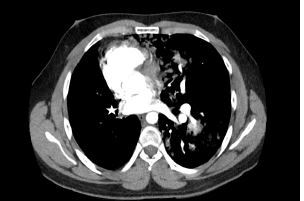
An axial view of a contrast-enhanced computed tomography angiography revealing an absent right pulmonary artery (star).
